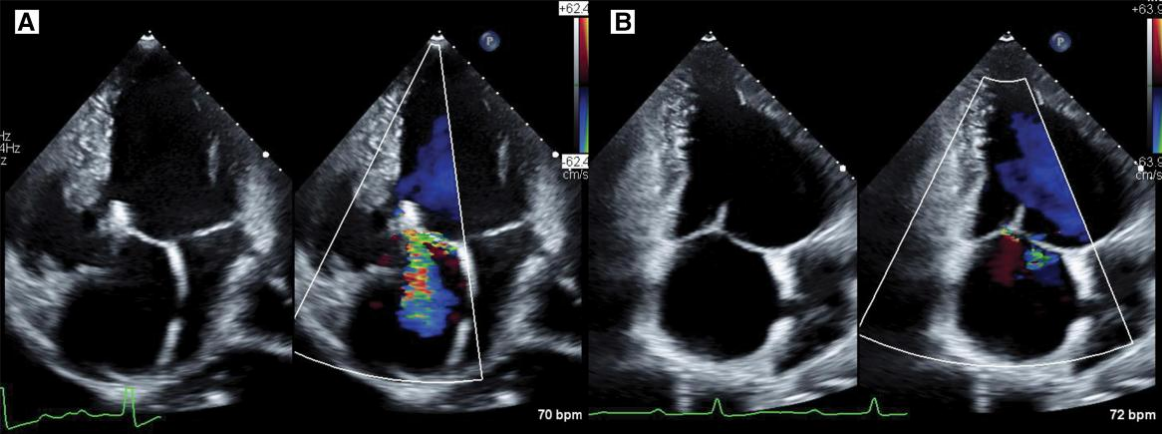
(*A*) Transthoracic echocardiography at discharge shows moderate tricuspid regurgitation. (*B*) Transthoracic echocardiography at the 1-month follow-up shows a decrease in the residual tricuspid regurgitation. TTE, transthoracic echocardiography; TR, tricuspid regurgitation.
